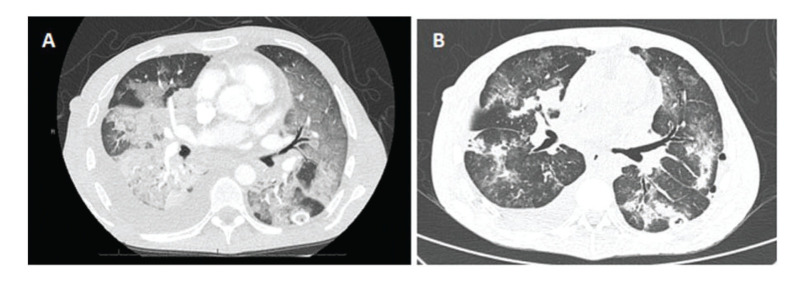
A) Chest computed tomography (CT) on post-operative day (POD) 12, one day before COVID-19 diagnosis, marked by bilateral alveolar opacities characterized as consolidation and ground-glass opacity areas covering more than 75% of the lungs B) Chest CT on POD 25 showing bilateral consolidation and ground-glass opacity areas under high resolution.
